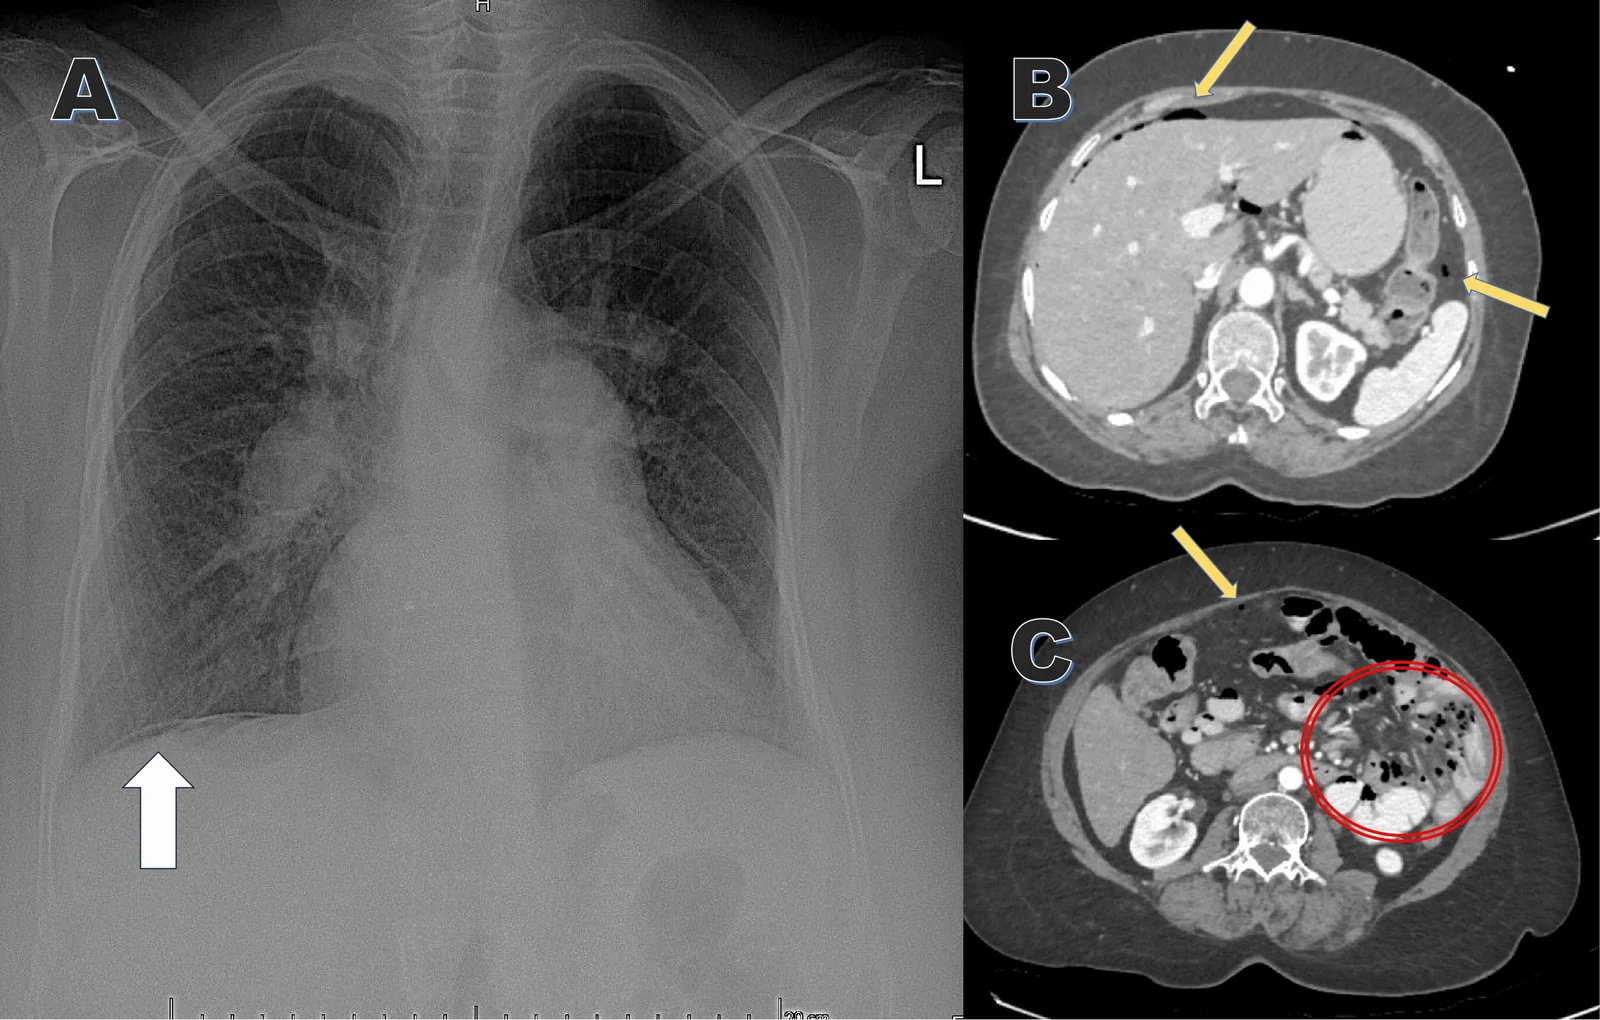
X-ray and CT images (A) X-ray study of the chest, posteroanterior (PA) view, showing subtle air under the right copula of the diaphragm (white arrow);  (B-C) Contrast-enhanced computed tomography (CECT) study of the abdomen with oral contrast, axial cuts, showing evidence of pneumoperitoneum (yellow arrows) and multiple jejunal diverticulae (red circle). No evidence of contrast leakage or signs of acute enteric obstruction.
